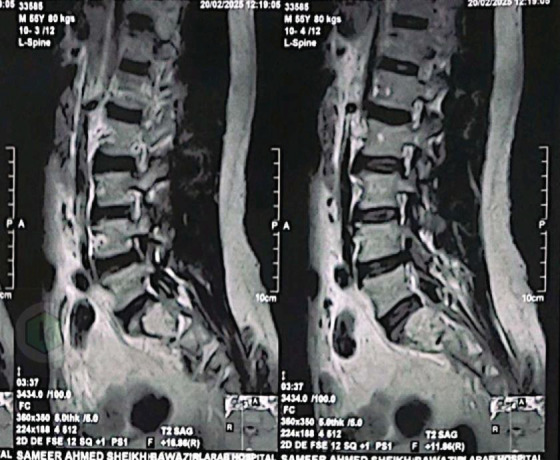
Sagittal T1-weighted MRI showing osteolytic sacral lesion (S1–S2).
